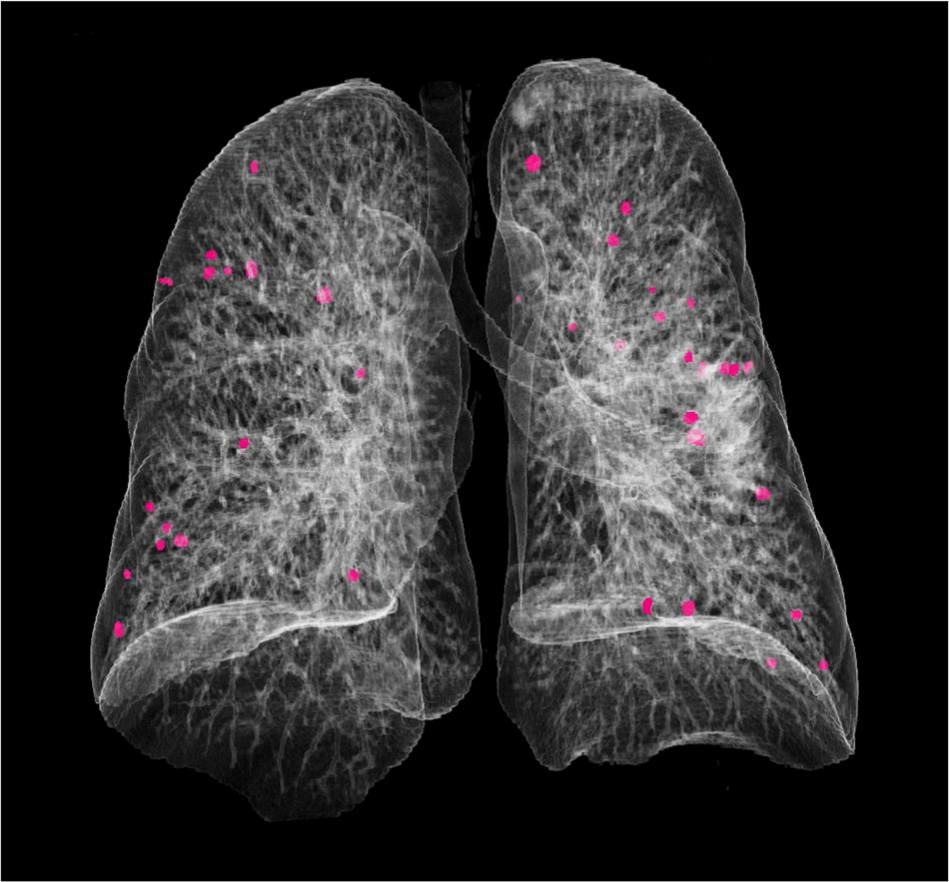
Nodule visualisation by computed tomography volume rendering. A three-dimensional (3D) volume rendering image from our patient demonstrates numerous pulmonary nodules (in magenta) with diameters between 3 and 12 mm (lung CT, 2022)
